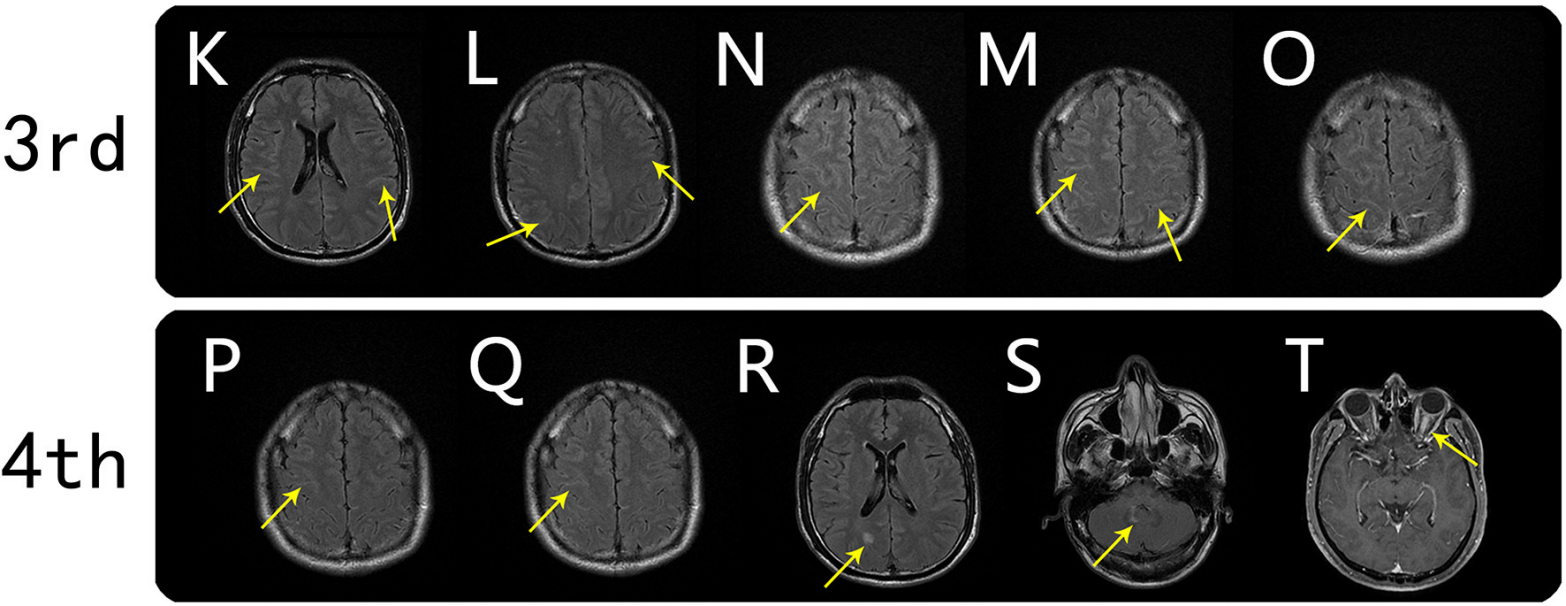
Brain MRI findings from the third and fourth scans. Third MRI scan (on day 22): axial FLAIR showed reduced meningo-cortical lesions that were still clearly observable (K–O, arrows). Fourth MRI scan (on day 37): axial FLAIR showed that the meningo-cortical lesions were further resolved (P and Q, arrows), but new lesions were present in the right parietal cortex and right cerebellar dentate nucleus (R and S, arrows). T1-weighted postgadolinium-enhanced image showing left optic nerve thickening and obvious enhancement (T, arrow).
